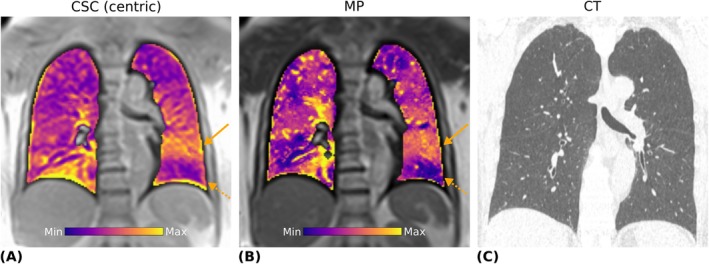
Fractional Ventilation of a 66‐year‐old female patient who underwent workup for a pulmonary nodule (not shown). Hypoventilated regions (dashed arrow) and hyperventilated regions (solid arrow) align in the CSC and MP analysis of 10 mm slice thickness. The ventilation maps were derived from the MP sequence (A) and the proposed CSC centric acquisition (B). The CT image shows normally appearing lung parenchyma (C).
